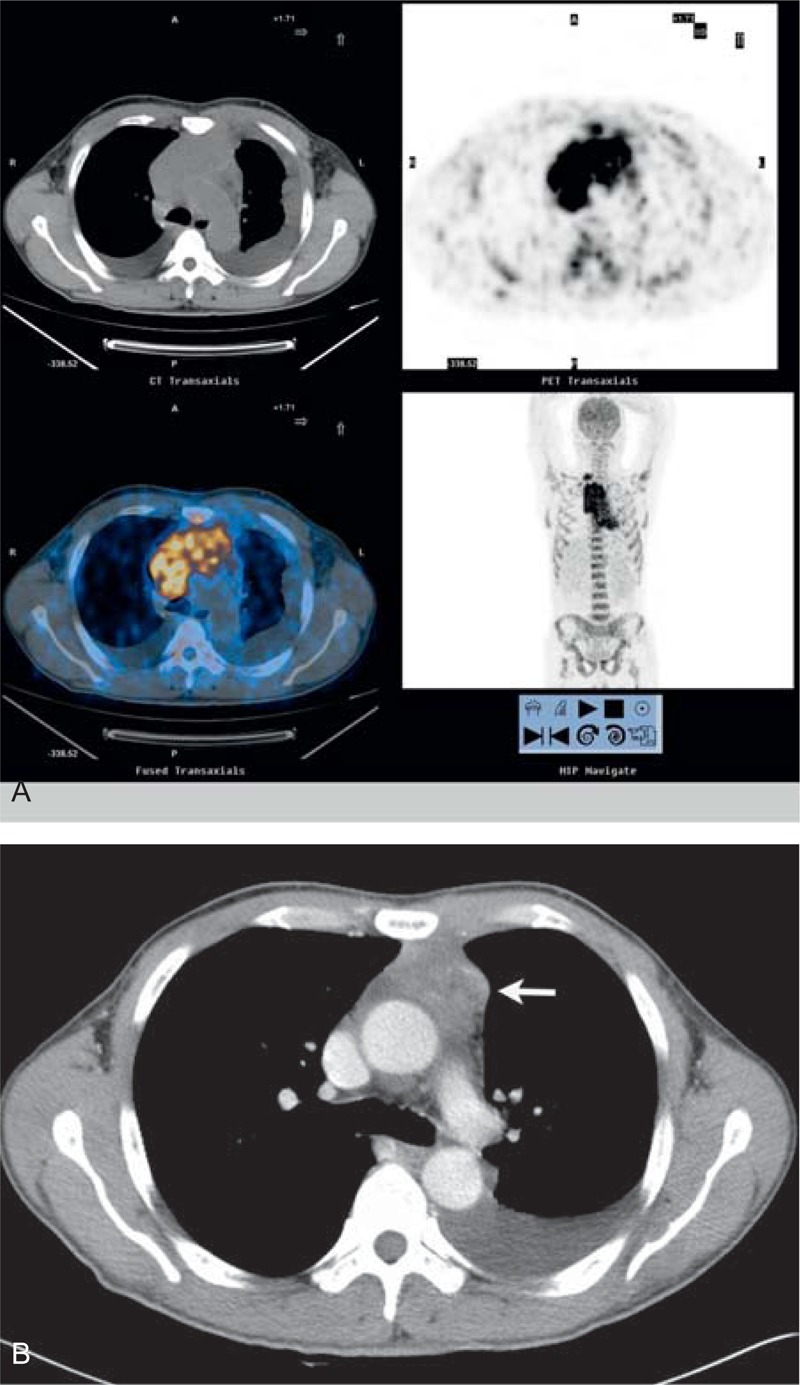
A 23-year-old man presented with a history of chest pain and shortness of breath for about 1 month. FDG-PET/CT images (A) show increased metabolic activity in the anterior middle mediastinal tumor, lymph nodes in the right supraclavicular fossa, and small bilateral pleural effusions; CT scan (B) shows that the tumor was remission at the 3-month follow-up after chemotherapy (arrow).
